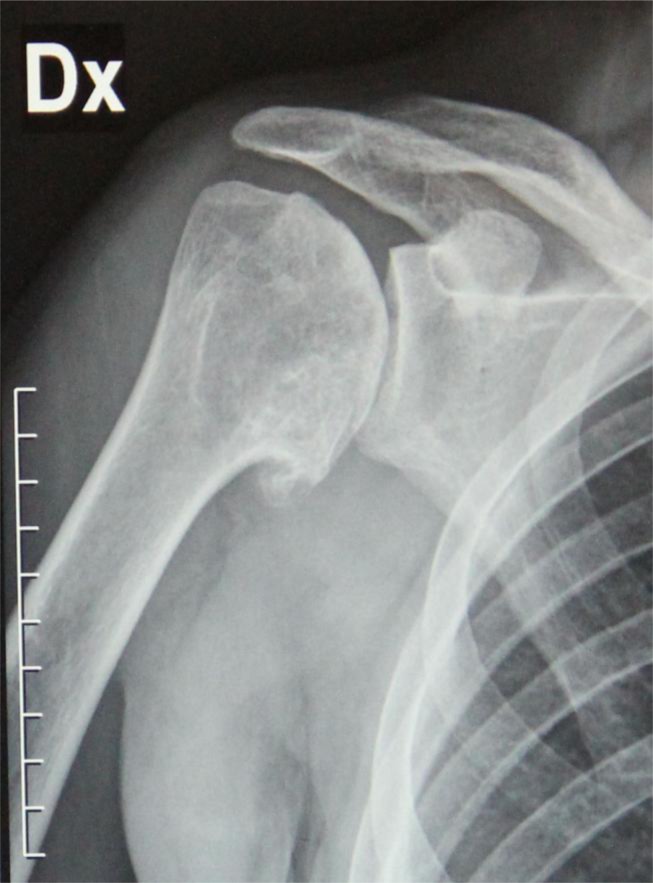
X-ray in true AP view shows severe gleno-humeral osteoarthritis with complete obliteration of joint space, glenoid erosion, and the humeral and glenoid osteophytes.
